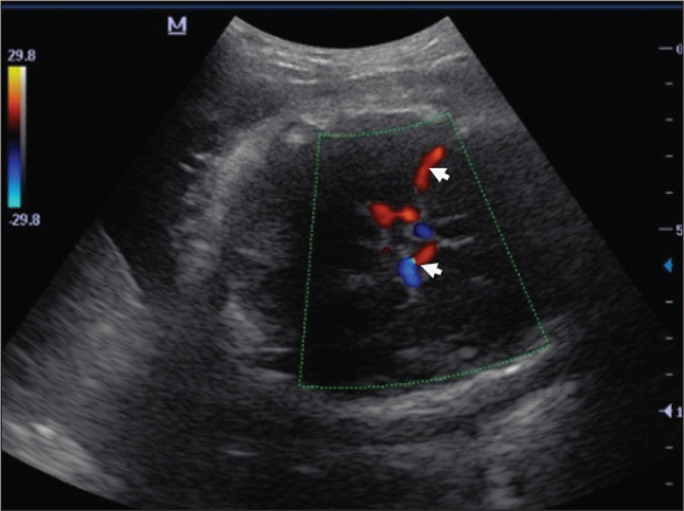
Color Doppler sonogram showing the fetal MCAs (arrows) in the Sylvian
